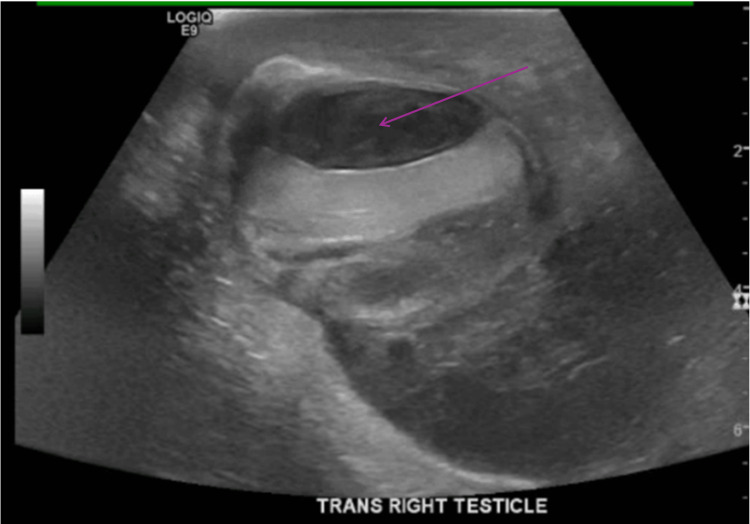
Ultrasound (transverse view) of the right scrotum The arrow shows complex mass/fluid collection.
